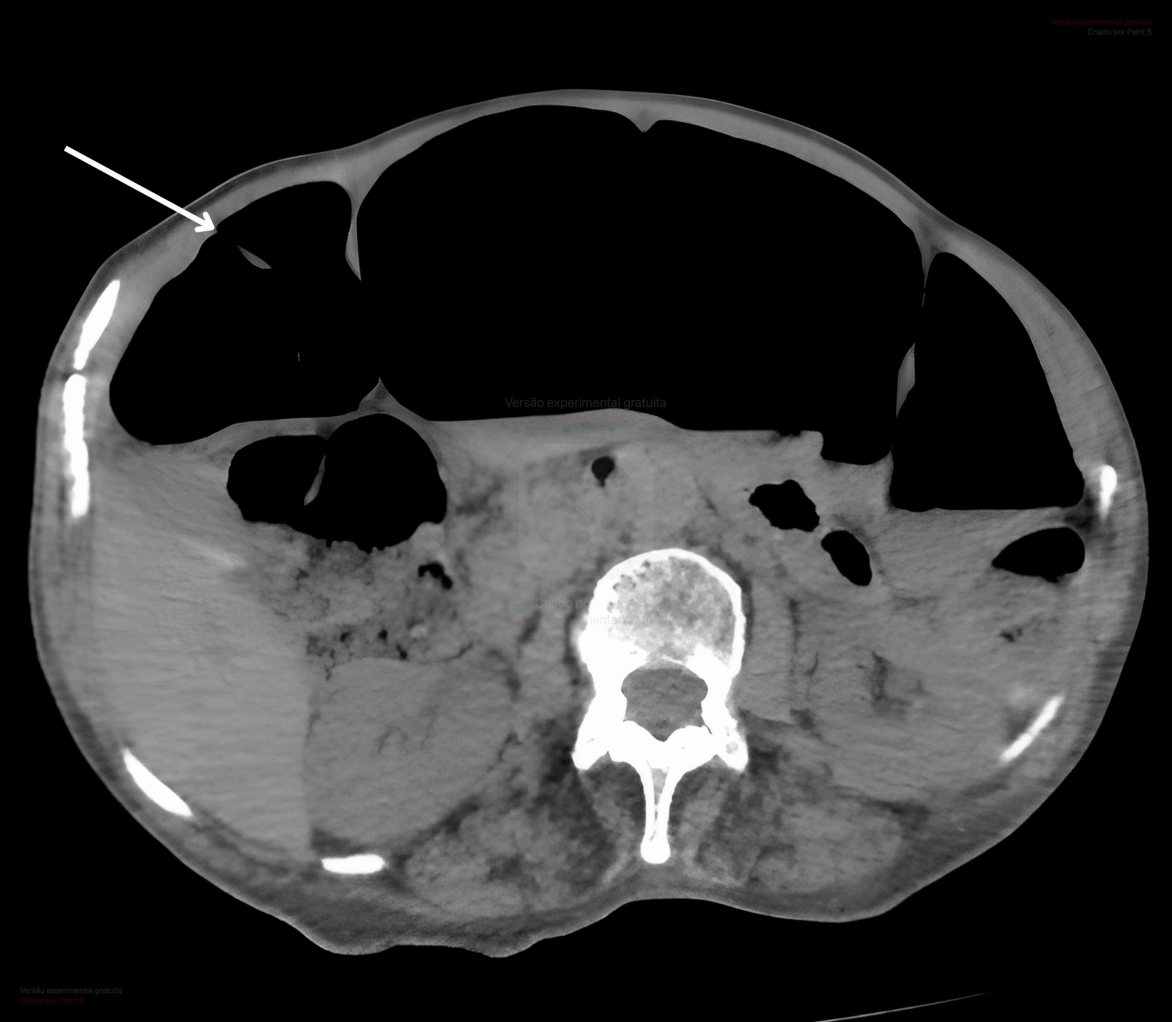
Abdominal CT showing colonic dilatation, predominantly involving the cecum and ascending colon, consistent with acute colonic pseudo-obstruction.
